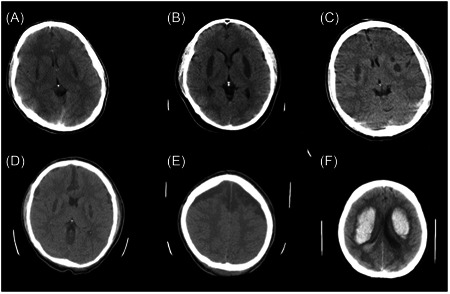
Brain CT scans can reveal the effects of methanol poisoning on the brain. The most common findings are hypodense lesions in the bilateral putamen, which may be symmetrical or asymmetrical (A–C). Few patients may also have subdural fluid collections, which are blood or cerebrospinal fluid accumulations between the dura mater and the arachnoid mater (D–E). These may compress the brain and cause neurological deficits or death. A rare but severe complication of methanol poisoning is hemorrhage in the putamen and the ventricles (F). This occurs from necrosis and rupture of the blood vessels in the affected areas. Patients with this condition usually have a low Glasgow Coma Scale score and seizures, and have a poor prognosis. CT, computed tomography.
